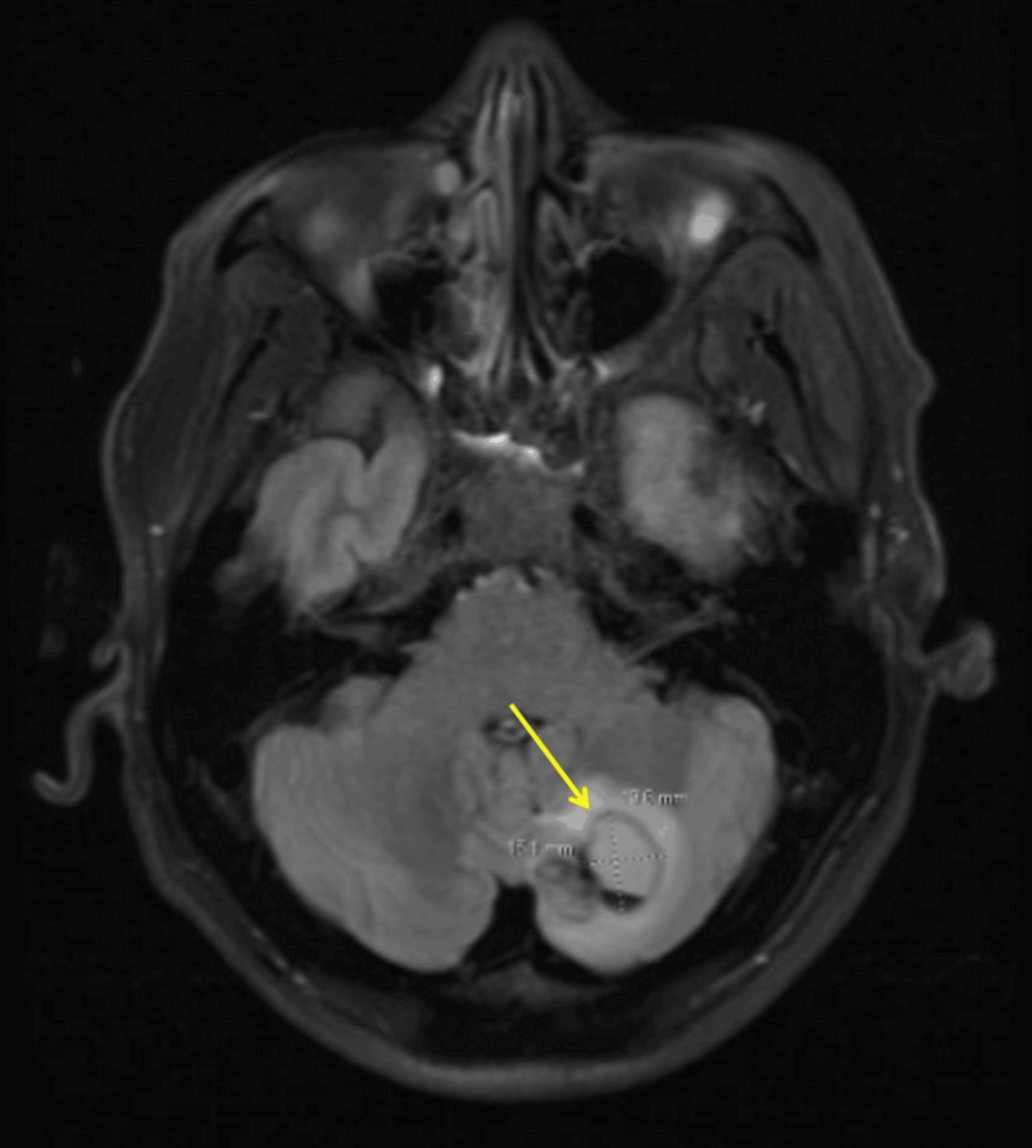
MRI of the brain revealed enhancing lesion in the left cerebellum (1.9 x 1.5 cm, arrow), suggestive of metastatic lesions. MRI, magnetic resonance imaging
